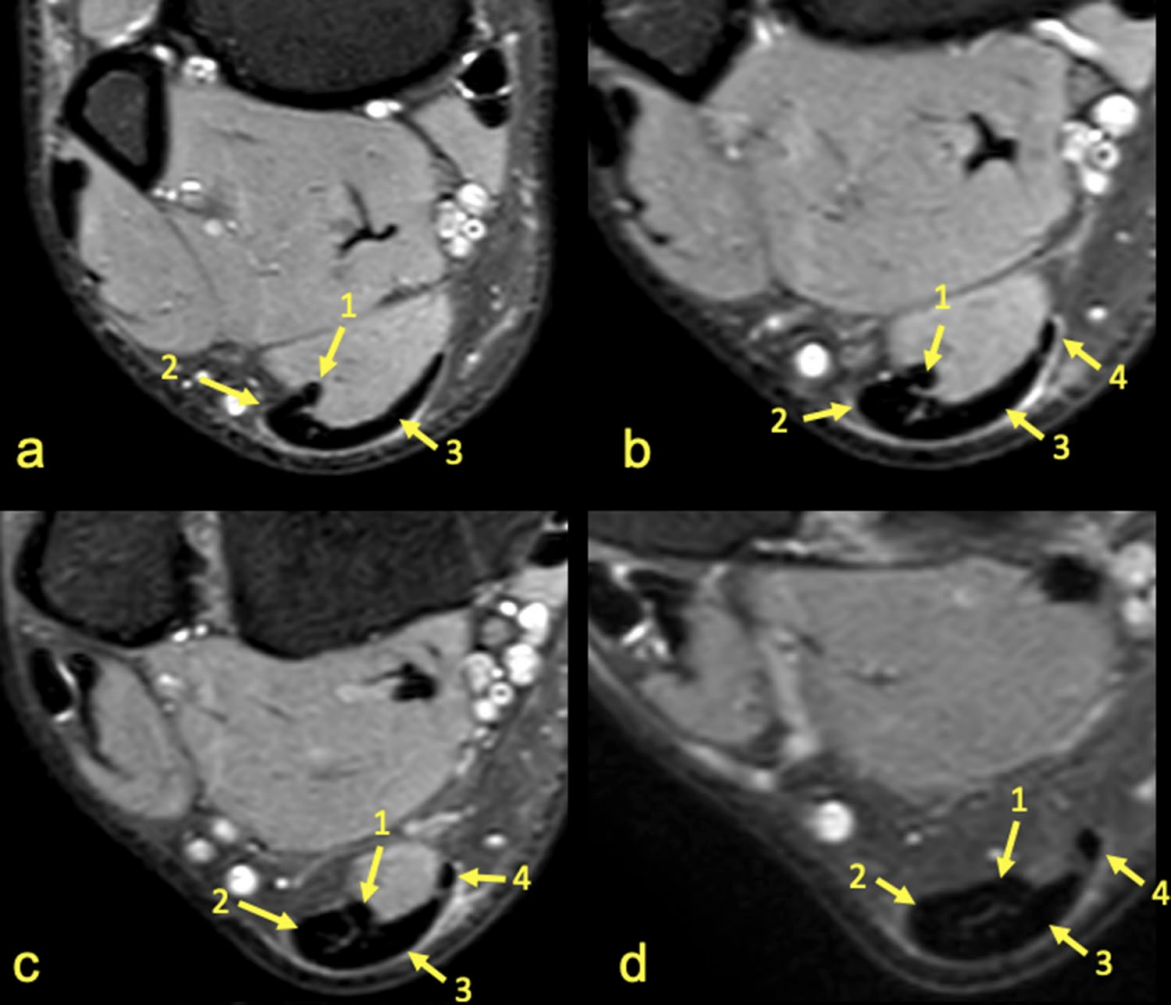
MRI right ankle, PD FS at the level of the distal tibia (a–c); (a)—the highest section, (c)—the lowest section, and the talus (d). A 39-year-old female 4 weeks after ankle sprain. 1—S-Sol, 2—S-LGC, 3—S-MGC, 4—the plantaris tendon (type 4).
